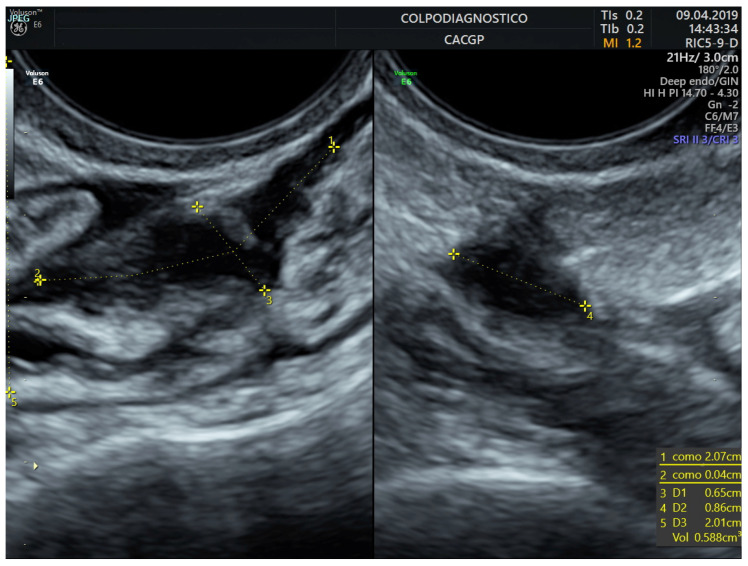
Transvaginal ultrasound image showing other measurements performed in an endometriotic nodule in the lower rectum.
